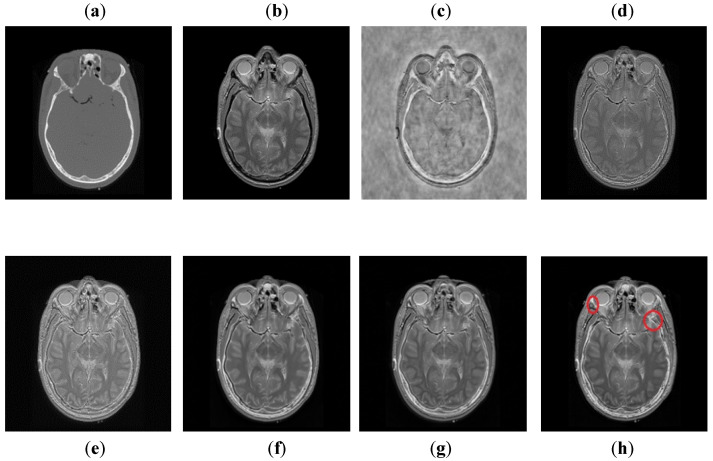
Data-4: (a) CT image, (b) MRI image, (c) DCT, (d) DWT, (e) NSST-SFT-PCNN, (f) CSR, (g) CNN, (h) and Proposed.
